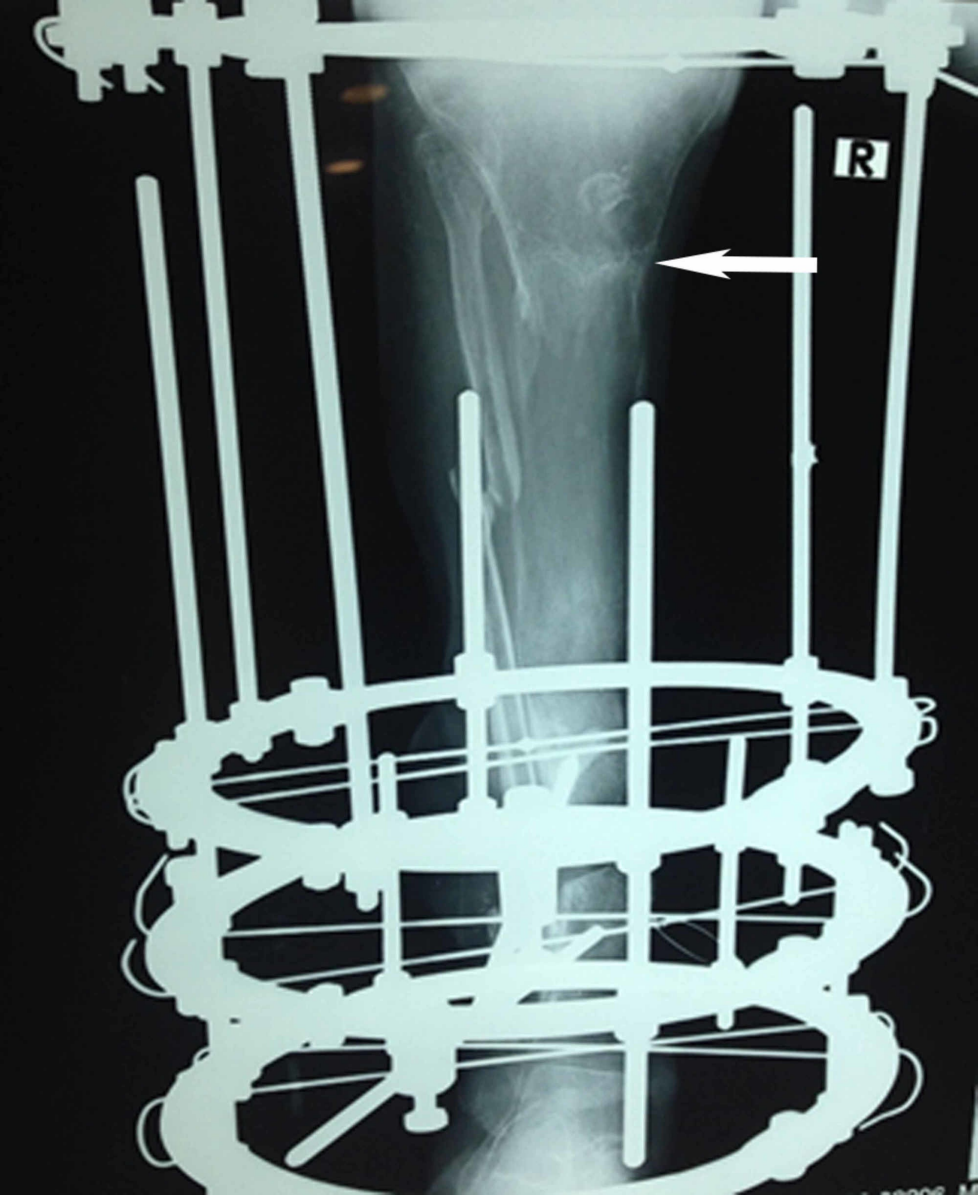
Postoperative plain radiograph of the same patient 13 months following the completion of bone transport showing the healed osteotomy site
